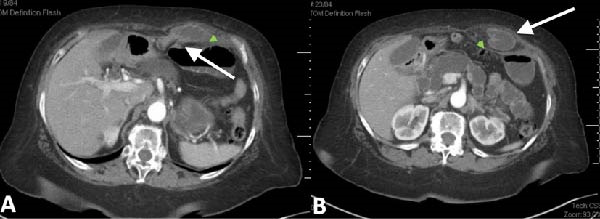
(A) CT images depicting neck defect in the posterior rectus sheath (white arrow) with surrounding rectus muscle (green arrowhead). (B) Herniated bowel (white arrow) through the posterior rectus sheath (green arrowhead).
